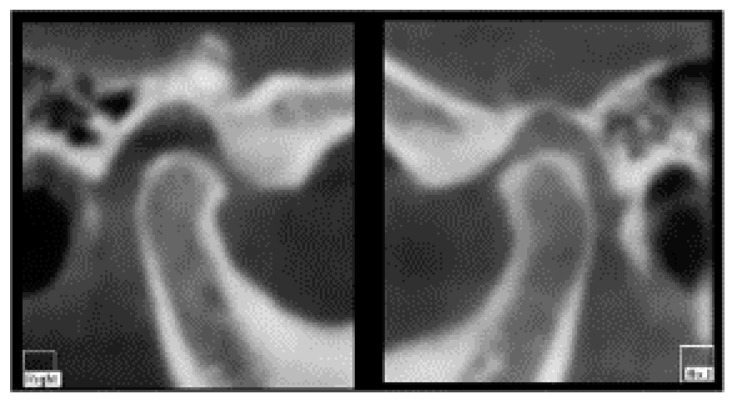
CBCT view of temporomandibular joints: no serious signs of morphological condylar involvement and a slight reduction of the intra-articular space.
